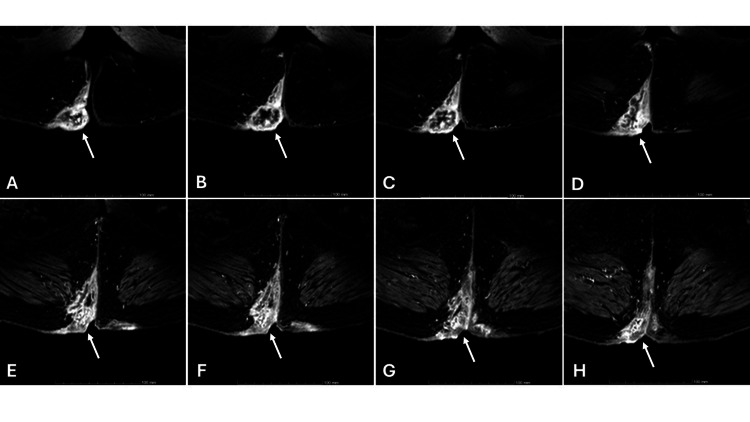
Pelvic magnetic resonance imaging of the patient described in the clinical case is shown in images A through H.
